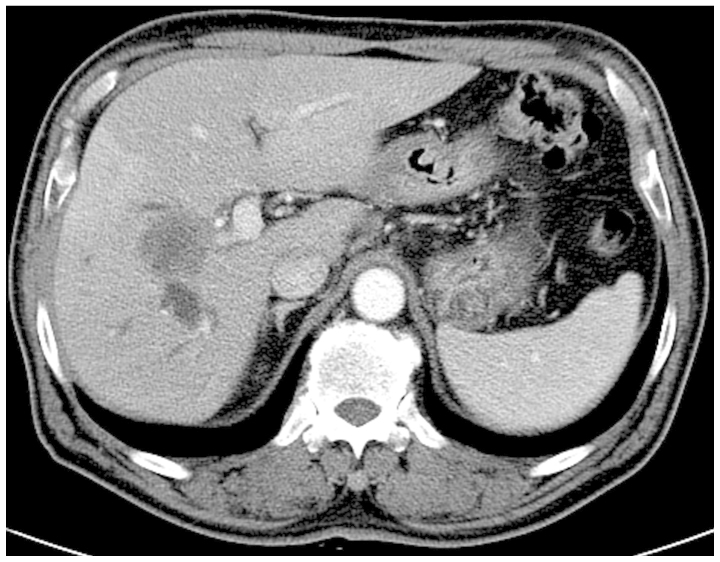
Computed tomography scan of abdomen following seven weeks of imatinib treatment. The previously noted hepatic mass had increased in size and the right intrahepatic duct dilatation was aggravated by the mass.
